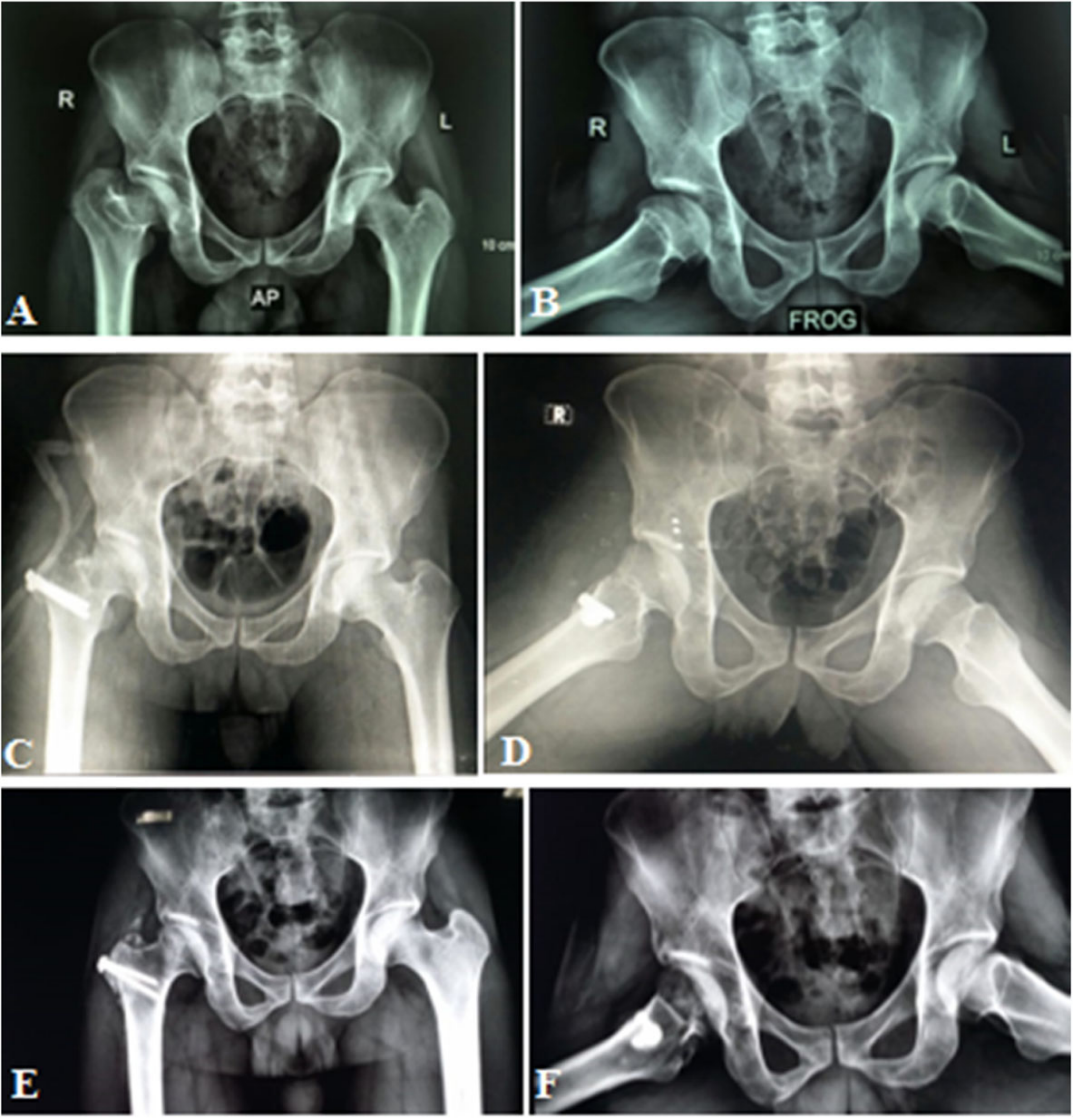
Radiographic evaluation of patient no. 10, preoperative (a, b), immediate postoperative (c, d), and 15 months postoperative (e, f)
